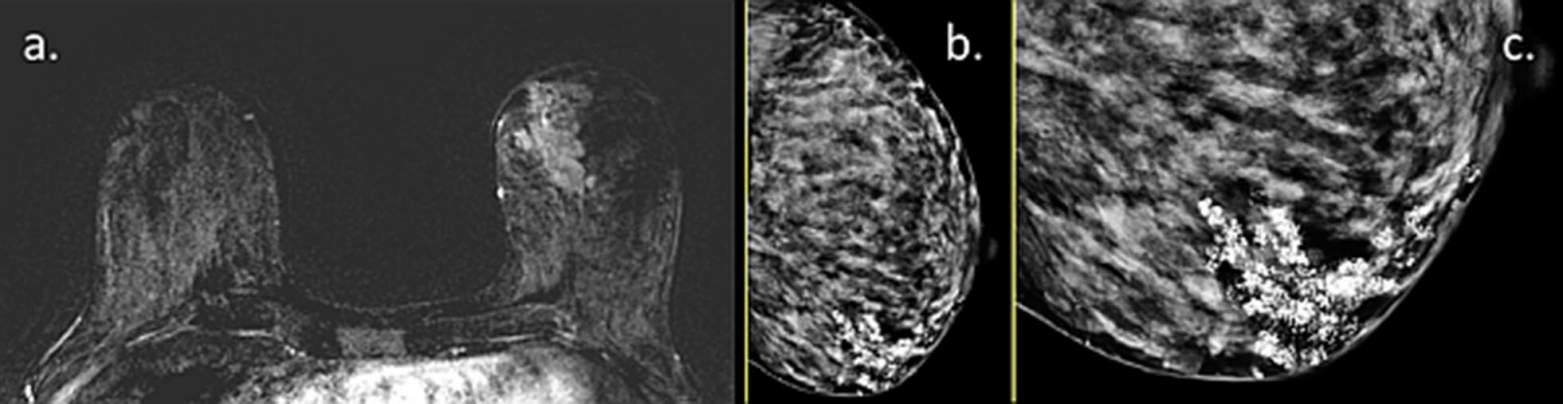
MRI of post-partum DCIS. Images of a 33-year-old PABC patient (lactating cessation a week prior to the MRI) diagnosed with left breast DCIS are presented. The patient palpate a lump in the left breast during the third trimester of pregnancy and underwent breast US (not presented) which depicted a benign appearing 9 mm oval mass. Upon follow-up 3 months later, post-partum, focal US depicted irregular mass with calcifications. Further diagnostic workup included US-guided biopsy, mammography which revealed extremely dense breast with segmental distribution of suspicious micro-calcifications in the inner-lower quadrant (b and zoomed image c), as well as breast MRI (a) which showed the characteristic NME of DCIS along 42 mm, in agreement with the mammographic findings, on top of the moderate background enhancement (BPE 2)
